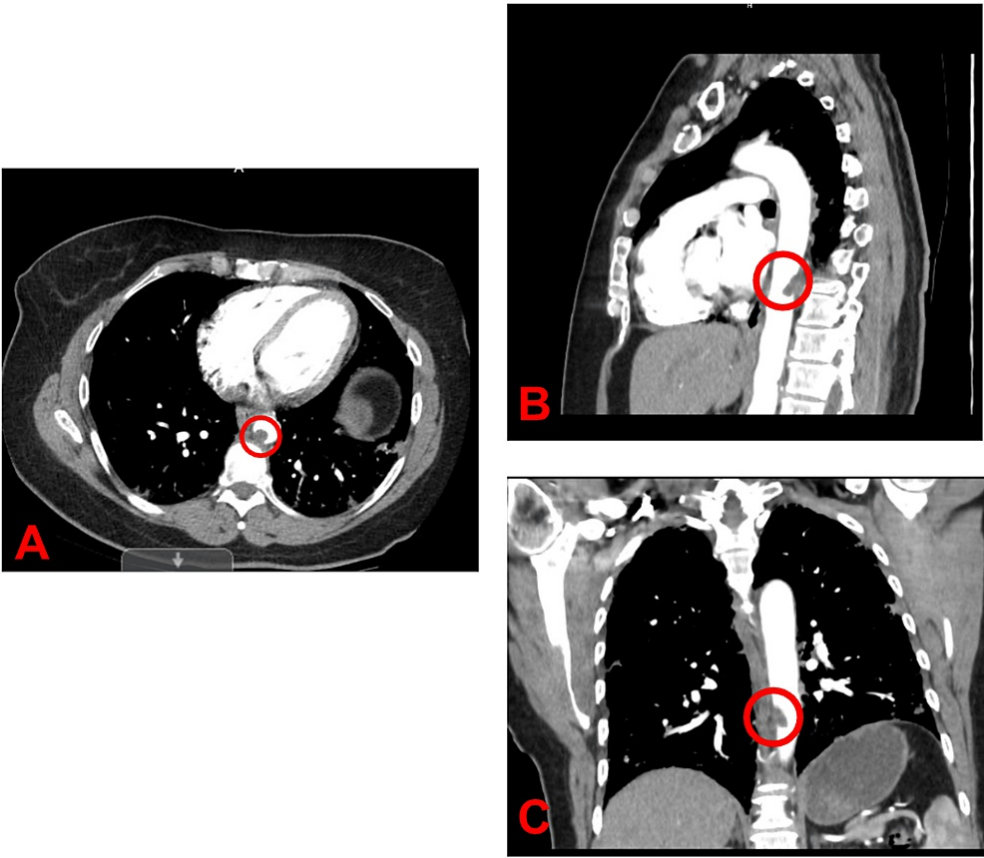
CTA of the chest showing pedunculated thrombus posteriorly in the descending thoracic aorta measuring approximately 1.7 cm in craniocaudal dimension and 1.3 cm in transverse dimension A. Axial view of CTA showing pedunculated thrombus. B. Sagittal view of CTA showing pedunculated thrombus. C. Coronal view of CTA showing pedunculated thrombus. The encircled region in A, B, and C indicates the pedunculated thrombus in the descending thoracic aorta. CT, computed tomography angiogram; AP, anteroposterior
